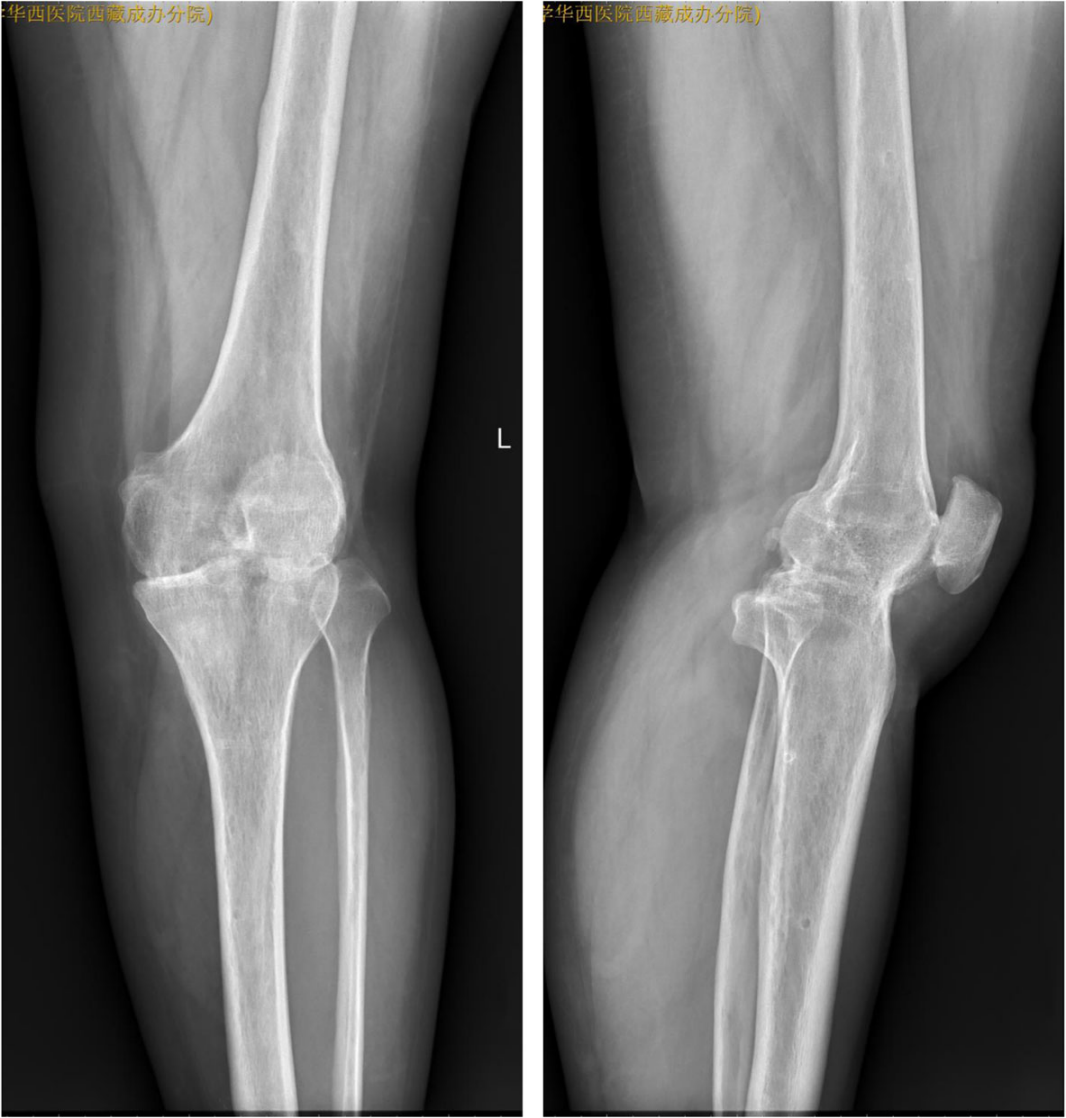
X-ray (anteroposterior and lateral): successful knee joint fusion (26 months after operation)
